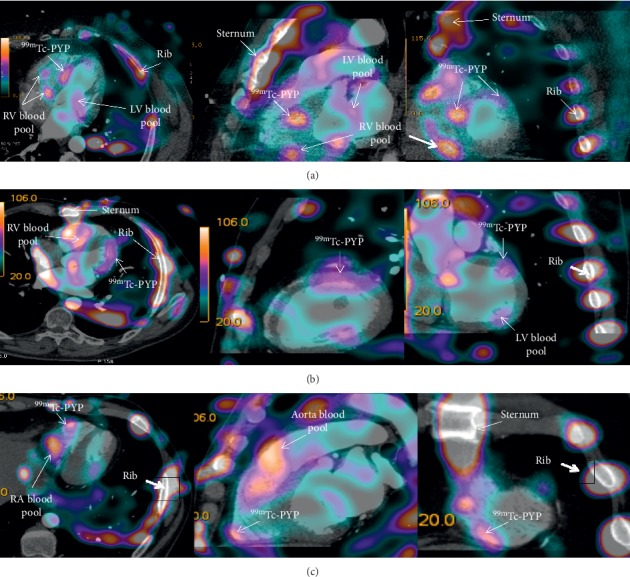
^99m^Tc-Pyrophosphate-based SPECT combined with multislice CT angiography. (a) Arrows show high-intensity focal accumulation of the radiopharmaceutical in the middle septal part of the left ventricle and low-intensity accumulation in the middle septal part and the anterolateral region of the left ventricle. (b) Arrows show diffuse moderate-intensity pathological accumulation of ^99m^Tc-Pyrophosphate in the middle basal part of the left ventricle. (c) Arrows show focal pathological accumulation of ^99m^Tc-Pyrophosphate in the middle part of the right ventricular free wall.
